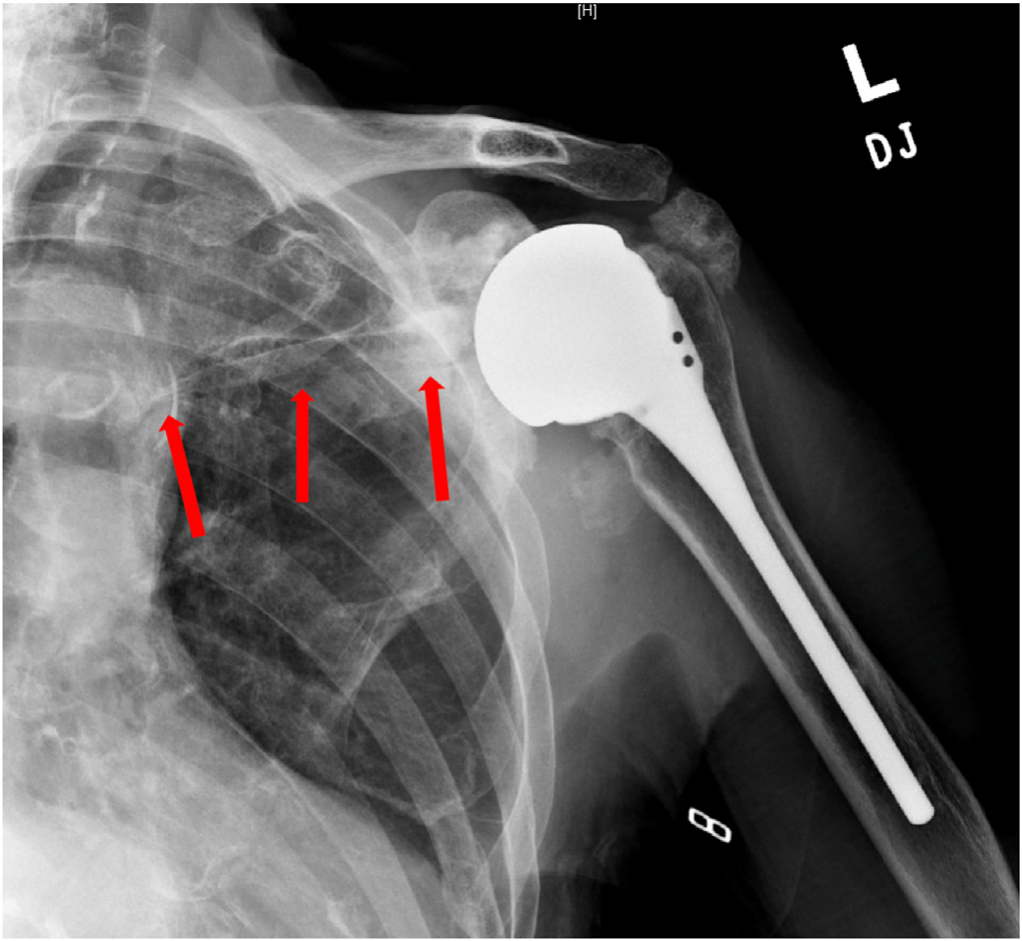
X-ray Grashey view demonstrating stable position of implant with signs of fracture healing.
